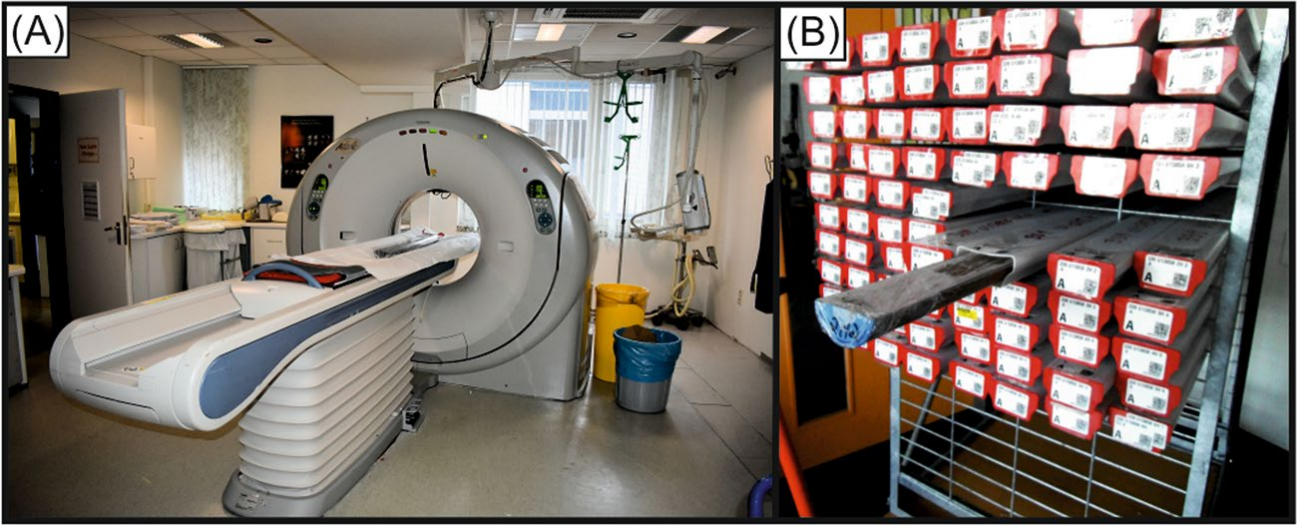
Computed tomography scan used in the present study (A) and some examples of the slabbed core sections from Site U1385 (B).
